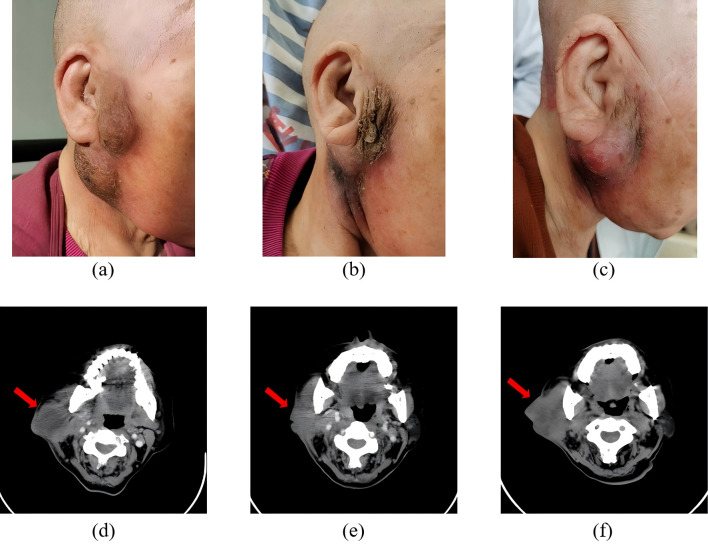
(A–C) show the physical examination findings of the patient on November 1, 2024 (pre-treatment), December 17, 2024 (post 2 cycles), and February 25, 2025 (post 5 cycles), respectively. (D–F) present local computed tomography images of the lesions obtained on October 30, 2024 (pre-treatment); December 17, 2024 (post 2 cycles); and February 26, 2025 (post 5 cycles), respectively. The lesion shrank markedly after 2 treatment cycles yet enlarged again after 5 cycles.
